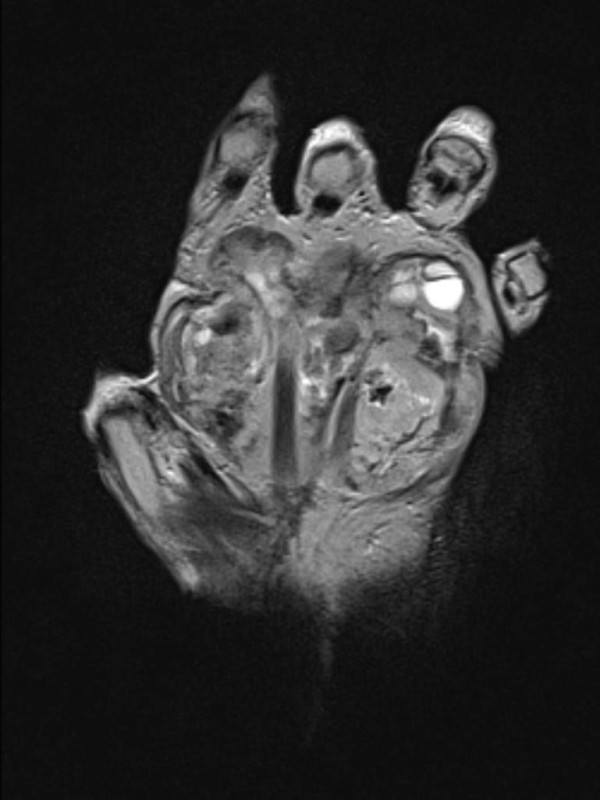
Magnetic resonance imaging scan of the hand showing tumor recurrence with a large hypervascular mass and foci of necrosis that occupied most of the anterior compartments of the hand and encroached into the metacarpal and carpal bones.
